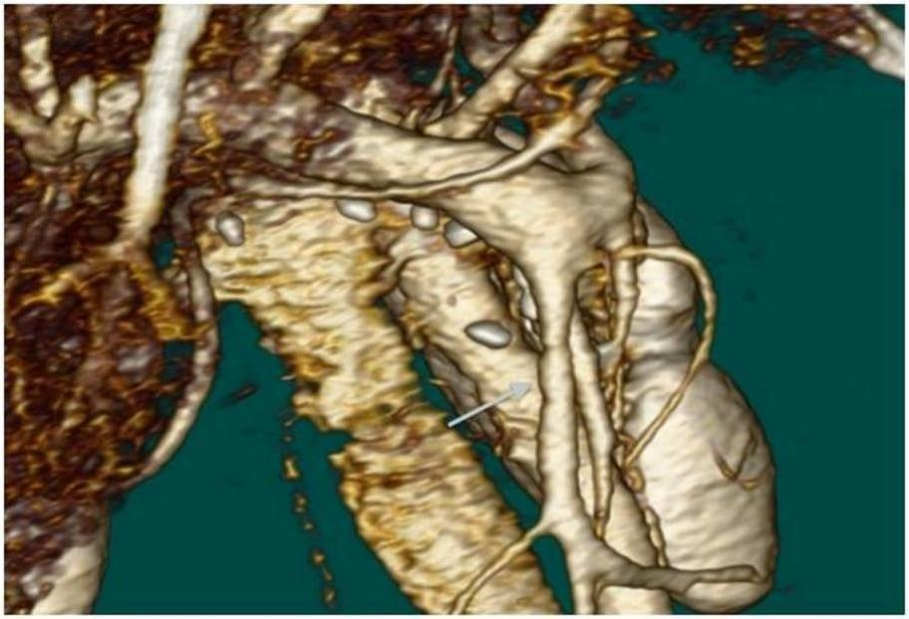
Contrast-enhanced 3D computed tomography of the patient on day 11. The superior mesenteric vein was patent.
